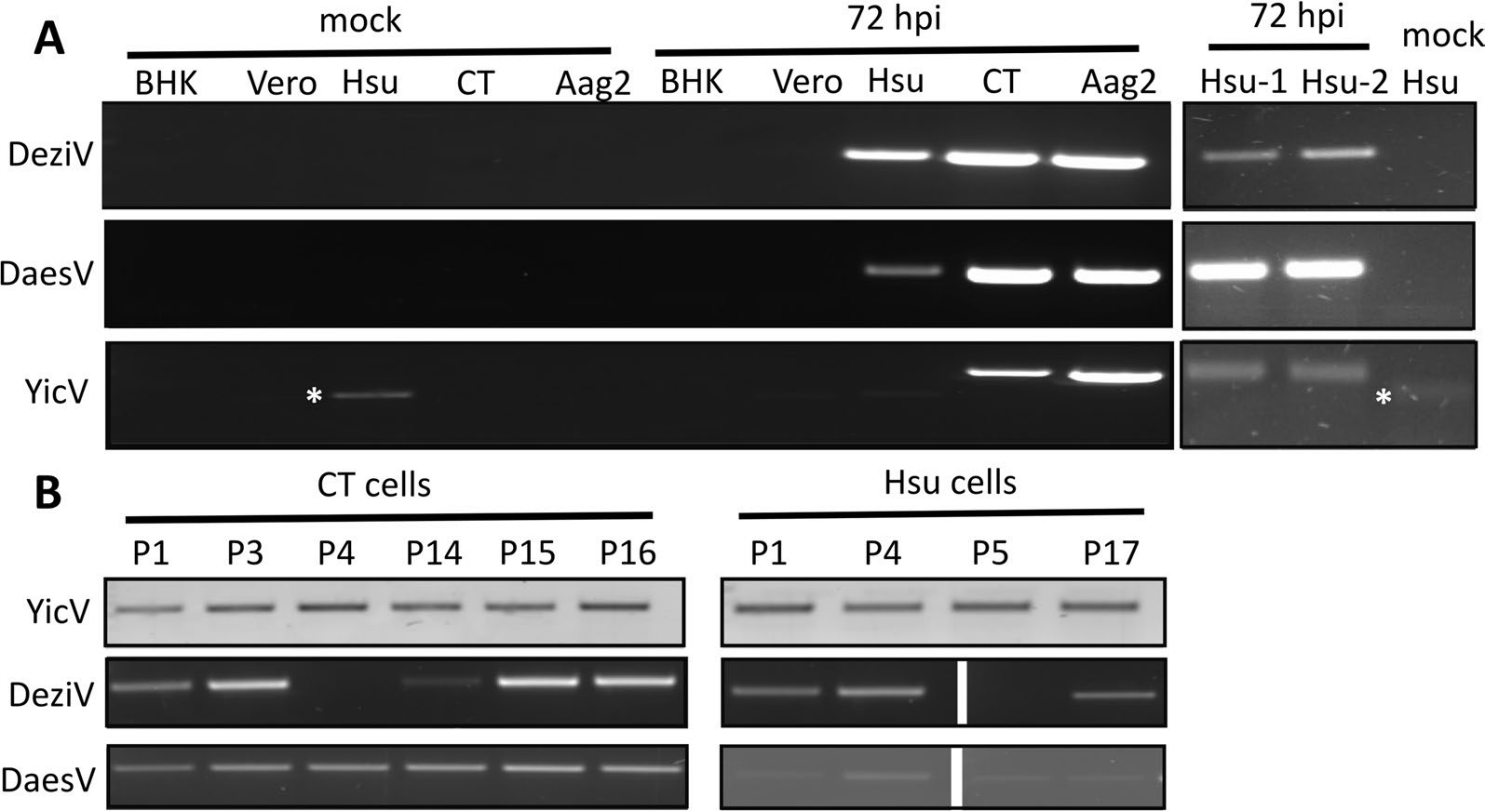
Mosquito-derived and mammalian cell lines co-infected with YicV/DeziV/DaesV. a Hsu (*Culex quinquefasciatus*), CT (*Culex tarsalis*), Aag2 (*Aedes aegypti*), Vero (*Chlorocebus* sp.) and BHK (*Mesocricetus auratus*) cells infected with the YicV/DeziV/DaesV mix (representative data of 2 independent repeats; except for Hsu cells). Right panel shows two additional repeats for Hsu-infected cells (Hsu-1 and Hsu-2, respectively). a, b The presence of YicV, DeziV and DaesV was detected by reverse transcription and virus-specific primers at 72 hpi (a) or at several passages for persistently infected CT and Hsu cells (b). The asterisk indicated an unspecific PCR band (verified by Sanger sequencing) in Hsu cells. DaesV, Daeseongdong virus (taxon Negevirus); DeziV, Dezidougou virus (taxon Negevirus); hpi, hours post infection; P, passage; YicV, Yichang virus (family* Mesoniviridae*)
